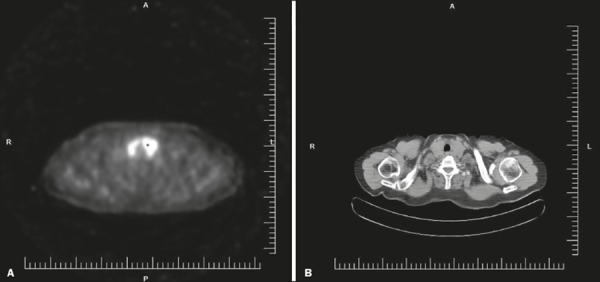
^18^F-FDG-PET/CT scan of a patient with primary thyroid lymphoma
